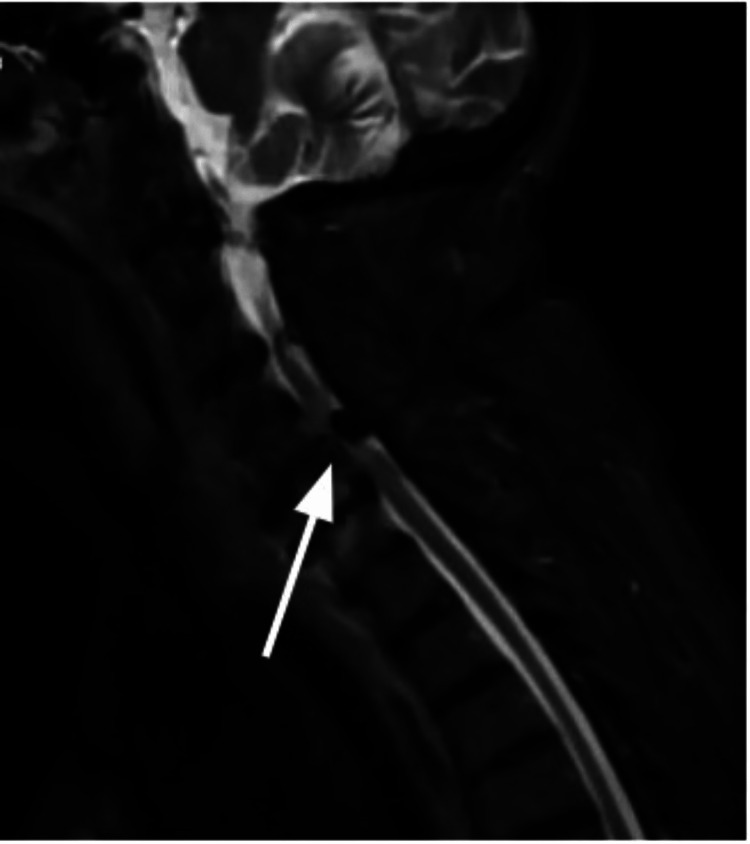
MRI cervical spine (sagittal) view of the cervical spine from admission demonstrating severe spinal canal stenosis predominantly in C5-C6 secondary to the anterolisthesis along with uncovering of the intervertebral disc and posterior facet hypertrophy. There is severe bilateral neural foraminal narrowing secondary to the anterolisthesis along with superimposed concentric disc bulge and osteophyte formation.
